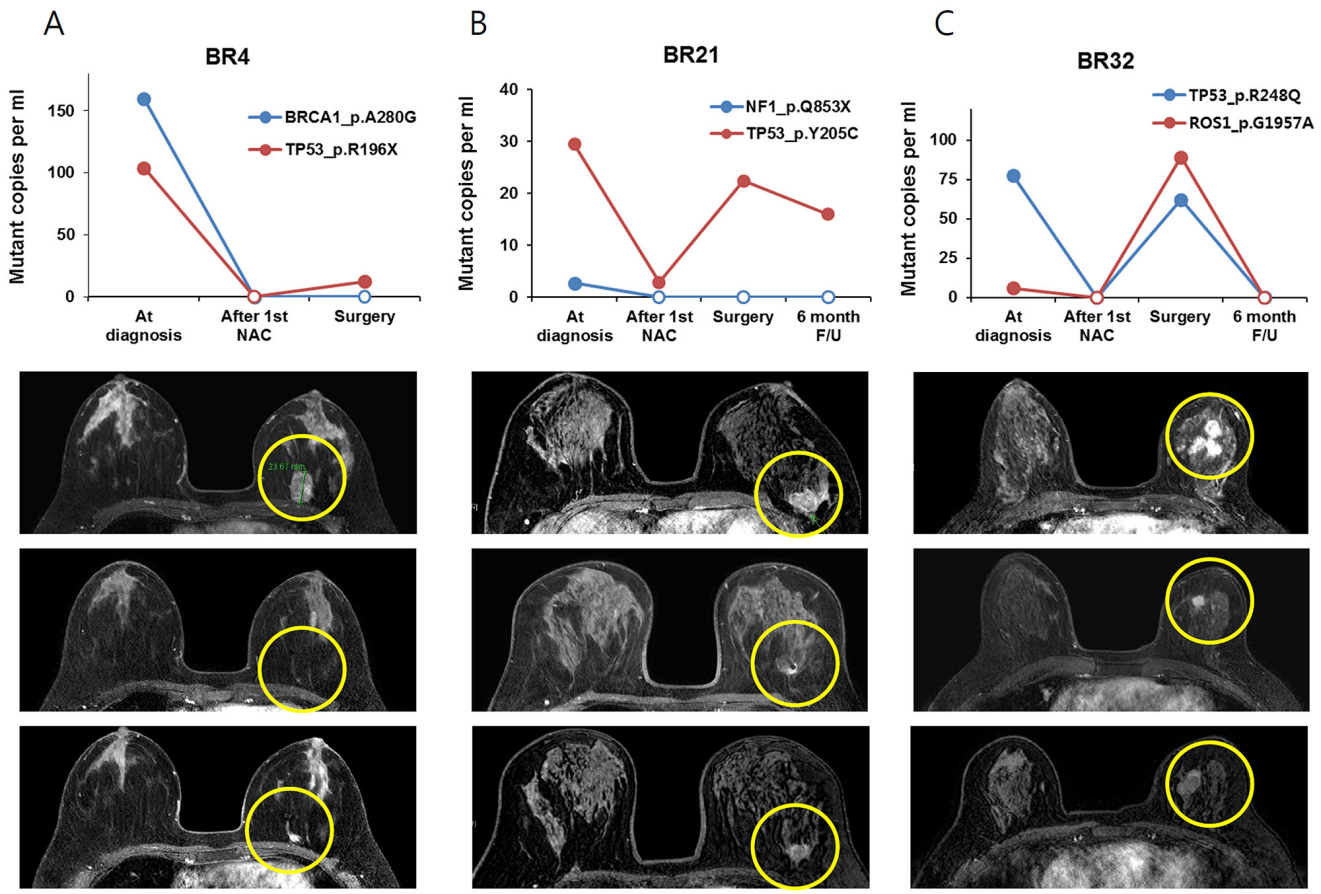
The change of ctDNA amount and MRI imaging in patients with residual tumor after NAC, tumor size decreased after the first 4cycles of NAC and then tumor size increased during the last 4cycles of NAC (A) BR4 (B) BR21 and (C) BR32 patients.
